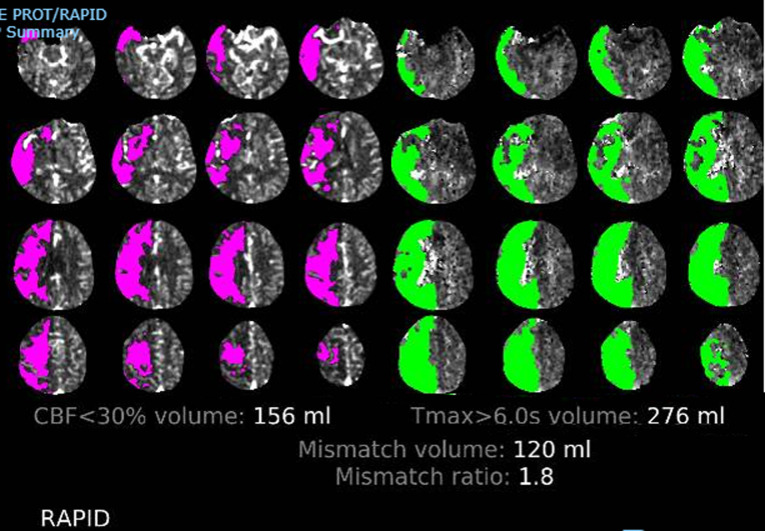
perturbation of the perfusion in the middle cerebral artery territory on brain computed tomographic perfusion sequence
